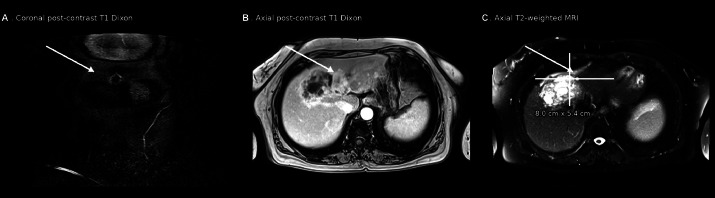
Final MRI examination (January 2025). (A) Coronal postcontrast T1-weighted image demonstrates marked interval reduction in lesion volume. (B) Axial postcontrast T1-weighted image demonstrates persistent benign enhancement characteristics. (C) Axial T2-weighted image confirms substantial spontaneous regression; white arrows indicate the residual lesion and measurement lines show transverse and anteroposterior dimensions of 8.0 × 5.4 cm.
